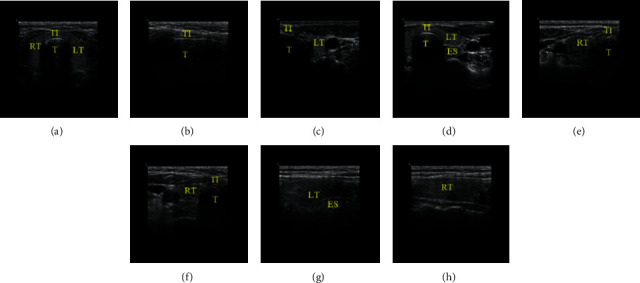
Thyroid Ultrasound Standard Plane images. (TI represents thyroid isthmus, LT and RT represent the left and right lobe of the thyroid, respectively, T represents the trachea, and ES represents esophagus). (a) TPTI, (b) LPTI, (c) UTPLT, (d) DTPLT, (e) UTPRT, (f) DTPRT, (g) LPLT, and (h) LPRT.
